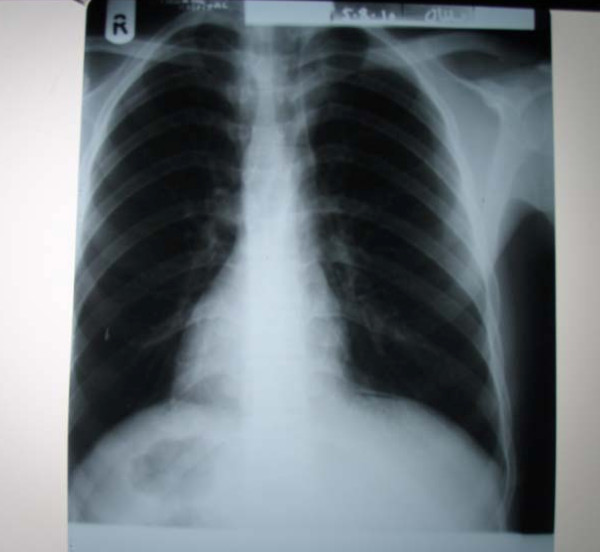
X-ray of the chest taken in the erect position, showing dextrocardia, fundic gas shadow under the right dome of the diaphragm, the liver shadow and free gas under the left dome of diaphragm.
